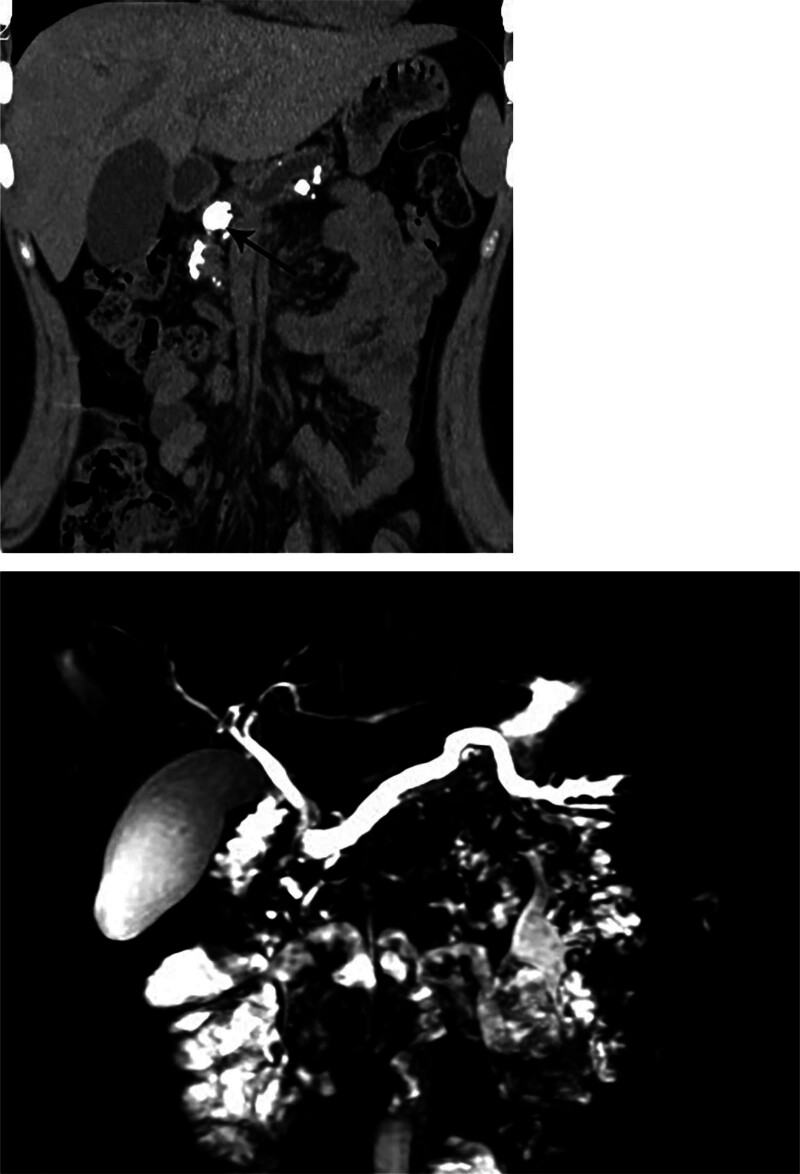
A computed tomography scan of the patient’s abdomen shows a dilated pancreas with an abnormally large stone embedded in the main pancreatic duct (indicated by an arrow).
